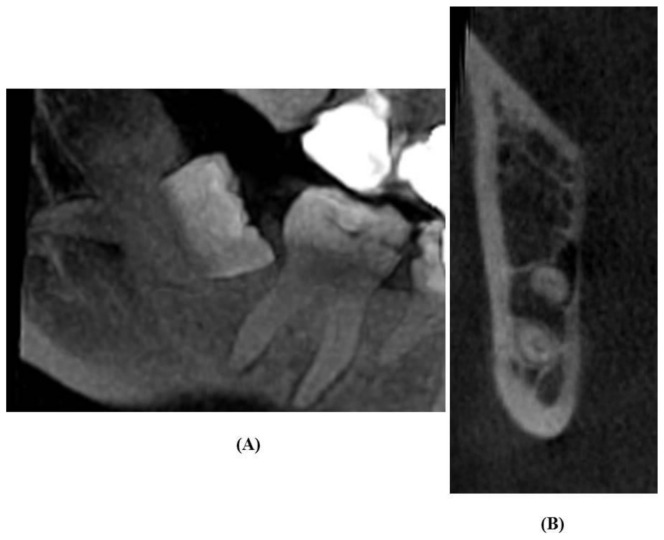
Panoramic images show darkening of the roots (A), and the inferior alveolar canal positioned between the roots without cortication on CBCT images (B).
